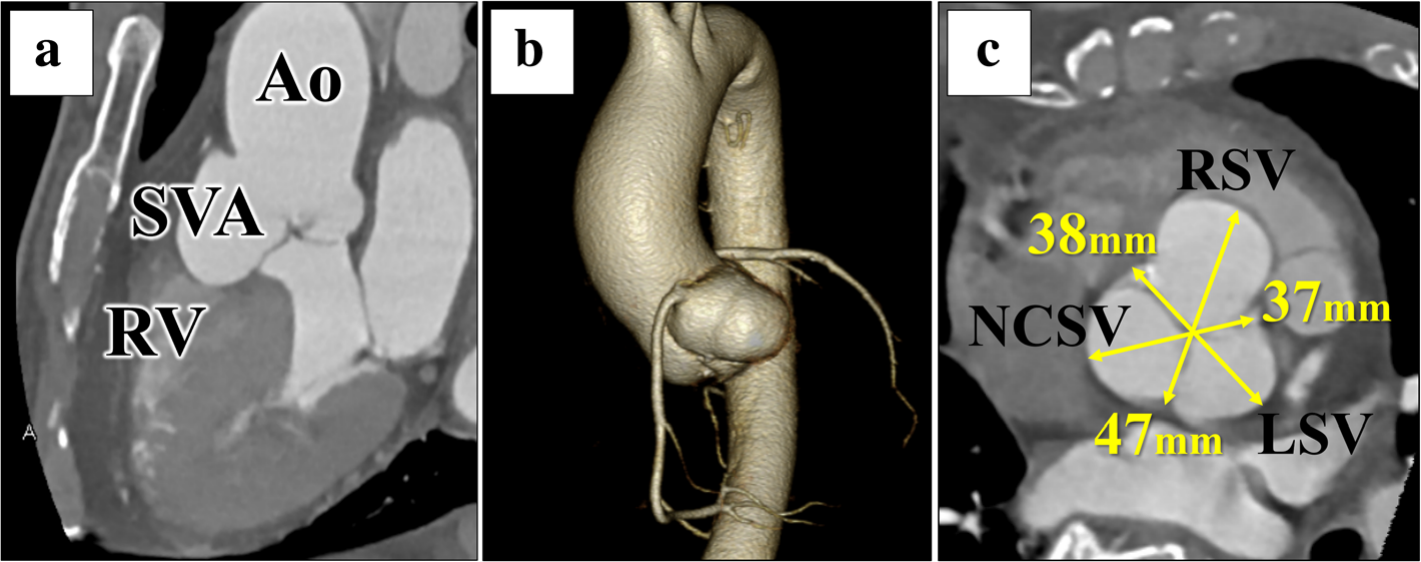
Preoperative computed tomography. a, b An unruptured right SVA protrudes into the right ventricular outflow tract. c RSV, 47 mm; LSV, 38 mm; NCSV, 37 mm. Ao: aorta; SVA, sinus of Valsalva aneurysm: RV, right ventricle; RSV: right sinus of Valsalva; LSV: left sinus of Valsalva; NCSV: non-coronary sinus of Valsalva
